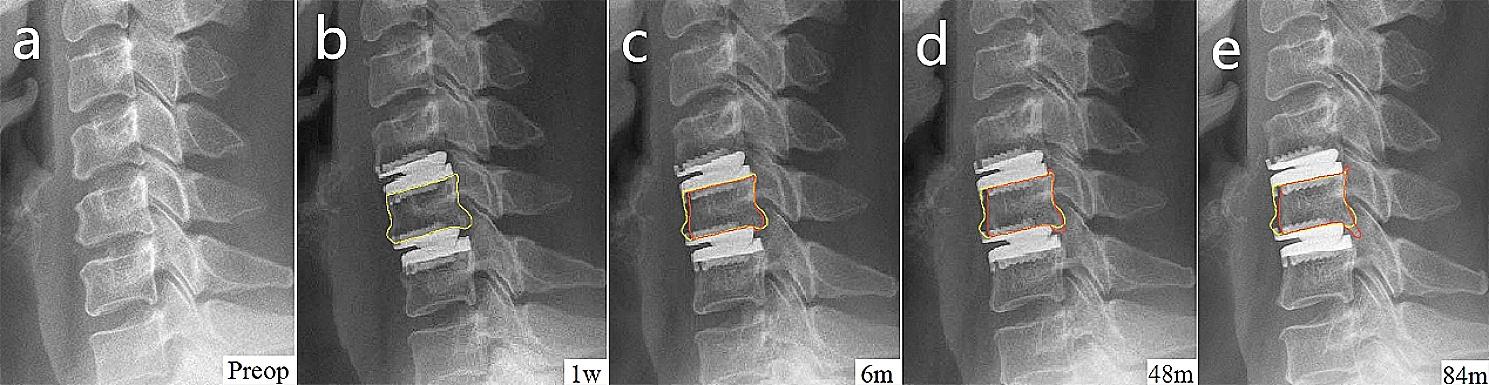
Serial radiographs of a representative case underwent contiguous two-level (C4/5 and C5/6) cervical disc arthroplasty. The series showed the sagittal area loss of middle vertebra. The changes in middle vertebral body profile are highlighted, with a yellow outline indicating the reference profile of 1-week post-operative and red outlines indicating the profile of each follow-up thereafter. a Pre-operative lateral radiograph of the cervical spine; b 1-week post-operative lateral radiograph showed cervical disc arthroplasty at C4/5 and C5/6 levels; c Early follow-up lateral radiograph showed moderate ABL at the anterior part of middle vertebral body; d and e Late follow-up lateral radiographs showed no obvious progress of anterior bone loss, but high-class heterotopic ossification developed at the posterior edge of caudal endplate. Sagittal area of C5 (follow-up time): 181mm^2^ (1 week), 172mm^2^ (6 months), 178mm^2^ (48 months), 179mm^2^ (84 months)
